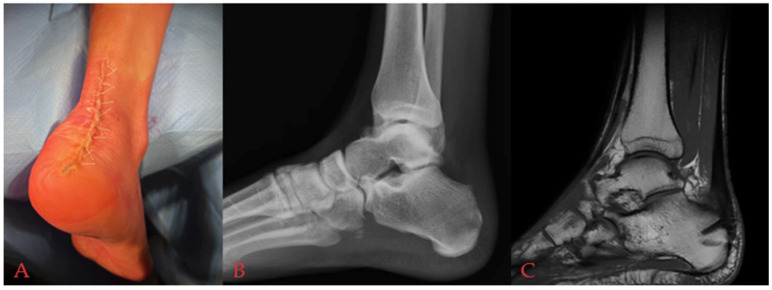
(A) Postoperative clinical image. (B) Postoperative X-rays. (C) Postoperative MRI.
